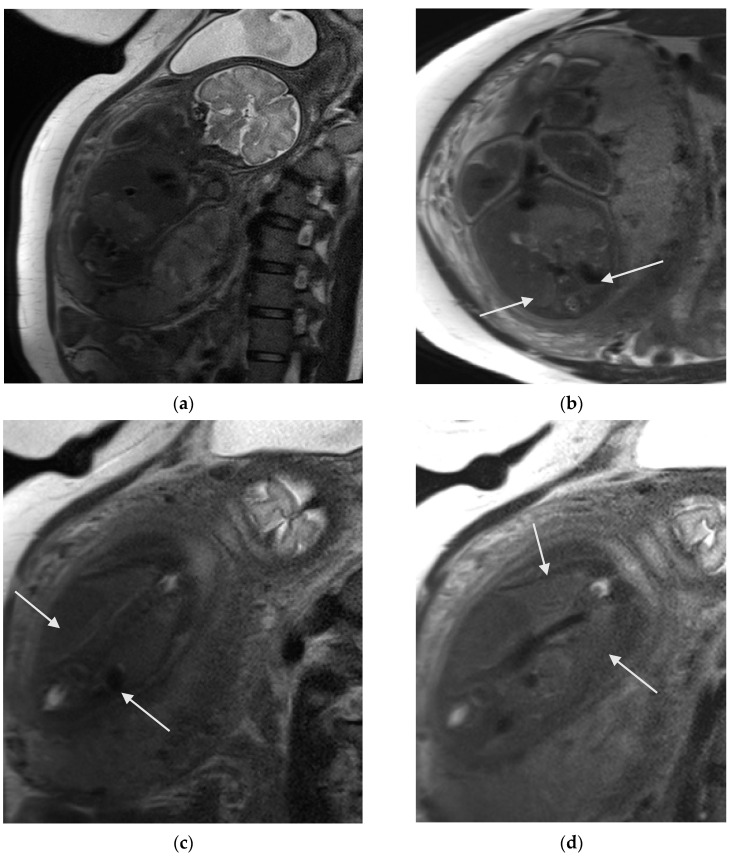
Fetal MRI at 32th week of gestation with bilateral renal agenesis. (a) Coronal T2W HASTE image shows severe anhydraminios. (b) Axial and (c) coronal T2W HASTE images show that bilateral renal fossae (white arrows) are empty, with no reniform structure identified. No fluid-filled structure in pelvis to suggest urinary bladder. (d) Coronal T2W HASTE image shows bell-shaped thorax (white arrows), suggestive of pulmonary hypoplasia.
